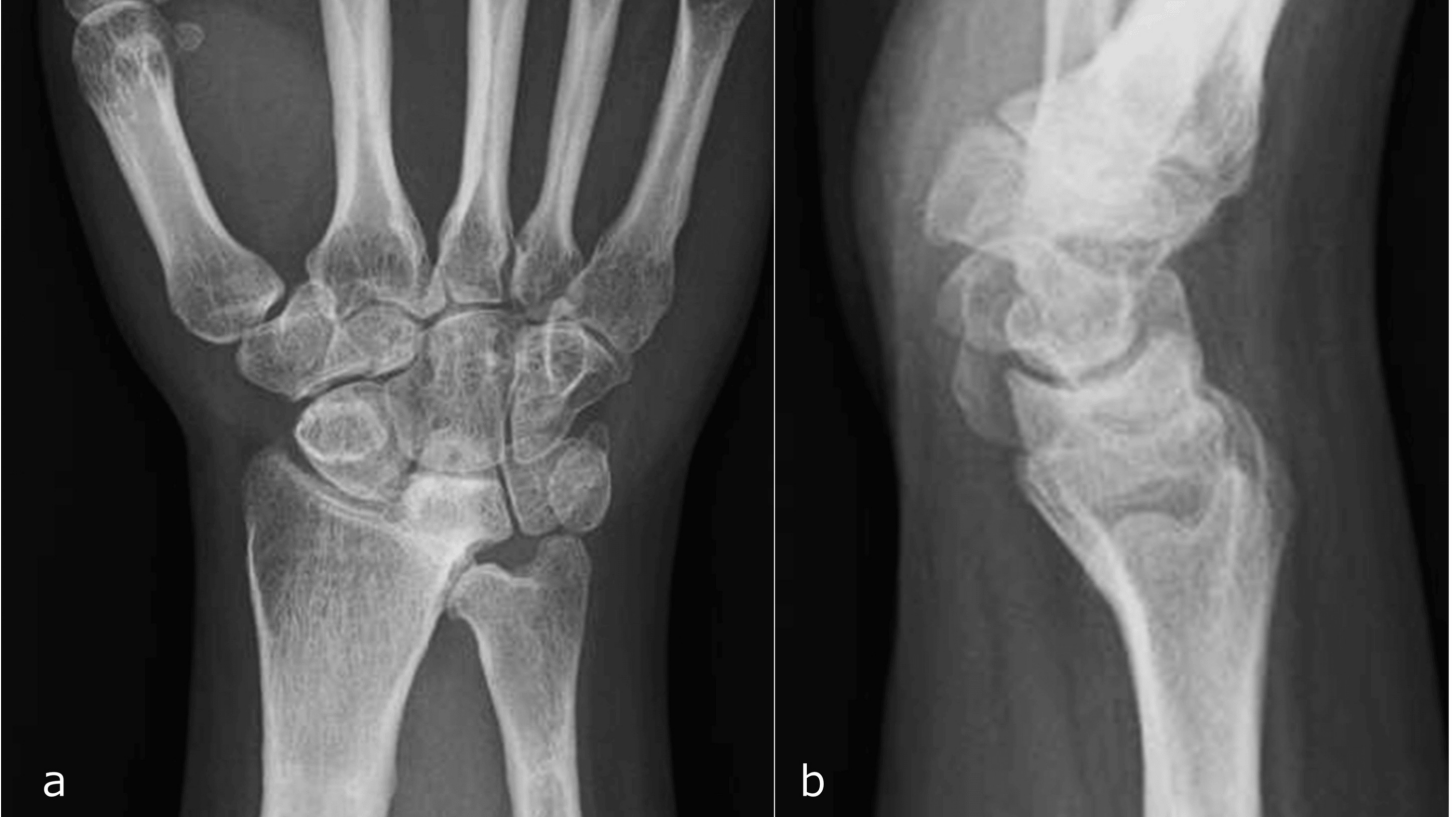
X-ray three years post-injury. a: Anteroposterior view. b. Lateral view.
